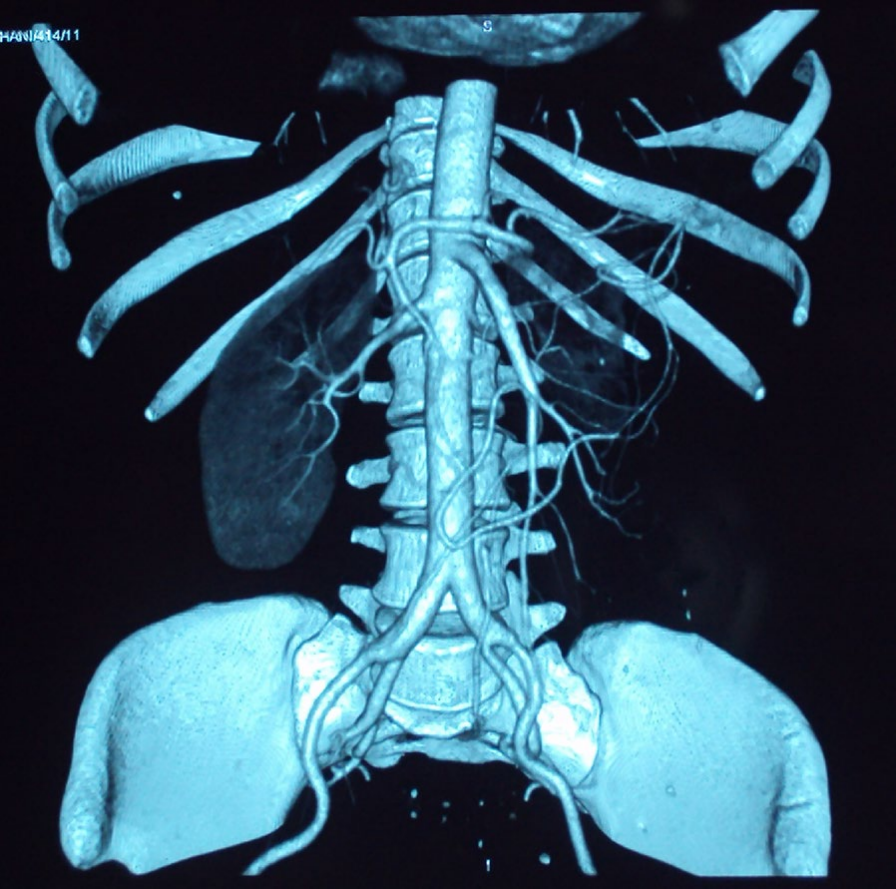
CT renal angiogram showing atrophic left kidney with non visualization of the left main renal artery
